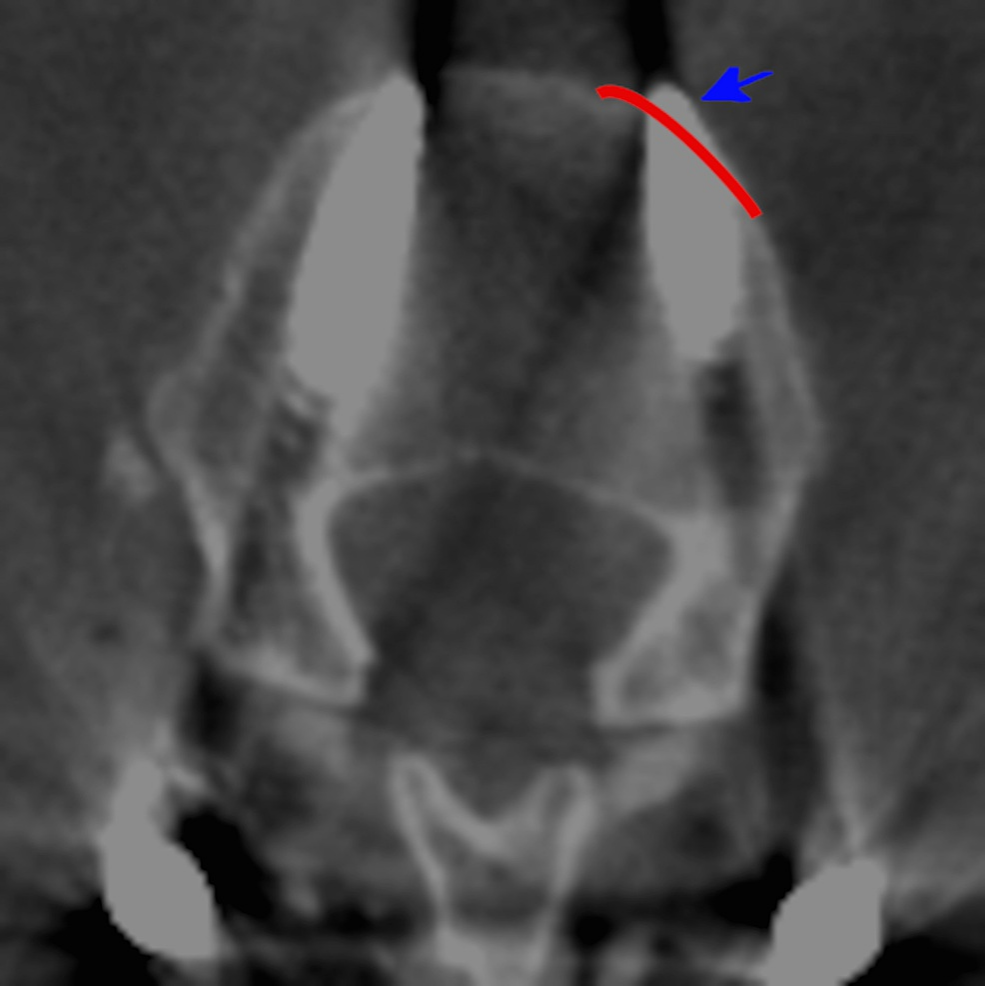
Axial CT radiograph of the left T12 anterior cortex breach on patient 10. An axial CT radiograph of the anterior cortex breach of the left T12 screw on patient 10. The anterior cortex is lined in red. A blue area denotes the screw deviation.
